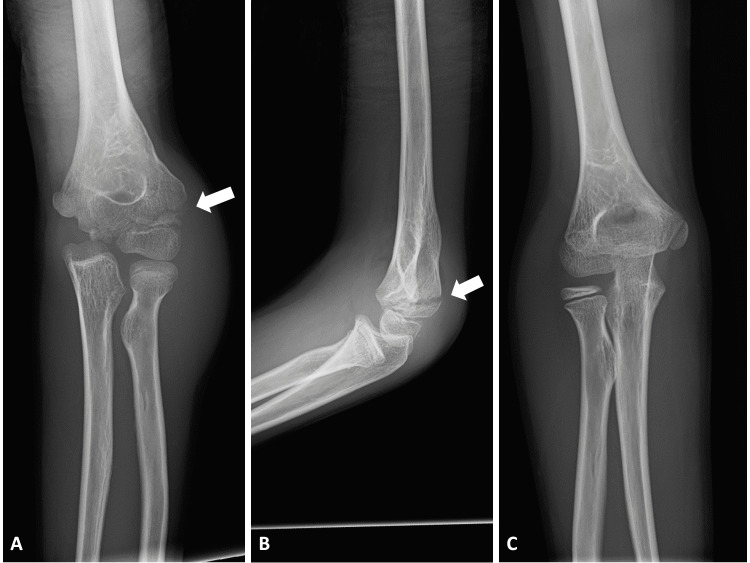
Plain radiography of the fourth fracture. (A) Anteroposterior and (B) lateral view of the left elbow. (C) Anteroposterior view of the uninjured right elbow. (Arrows: fracture site).
